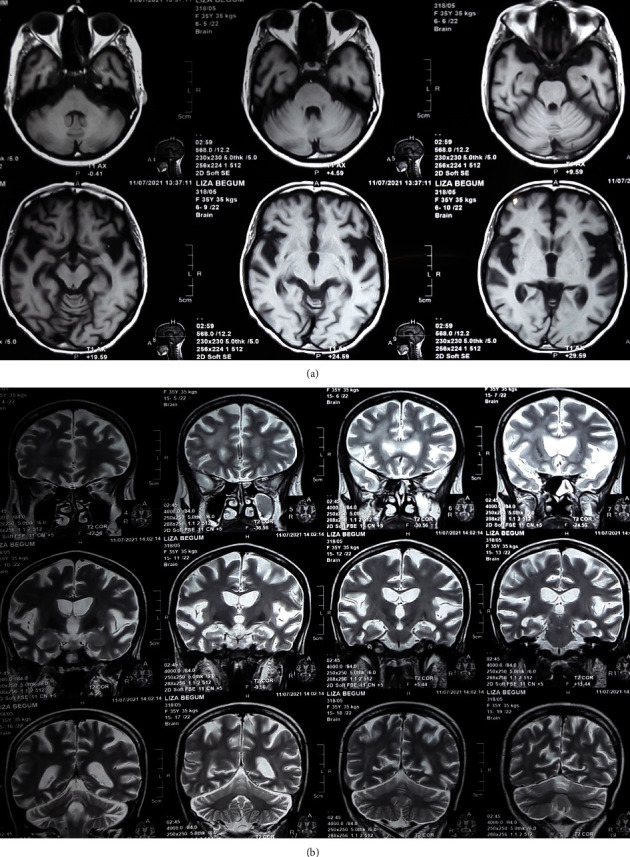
MRI brain: (a) T1 axial view and (b) T2 coronal view, showing generalized brain atrophy.
